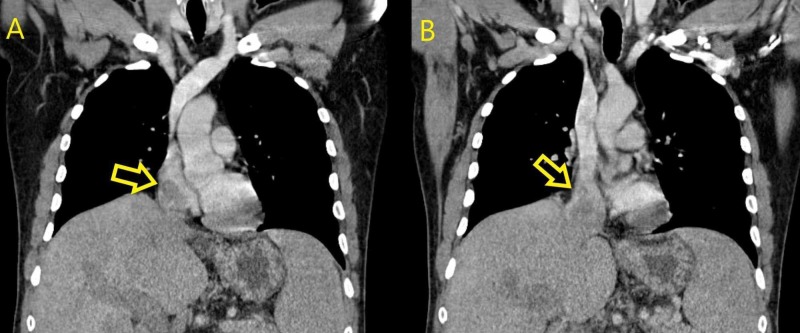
Coronal view of CT scan of the chest showing: A & B: Tumor thrombus making its way through the IVC to the right atrium CT, computed tomography; IVC, inferior vena cava
